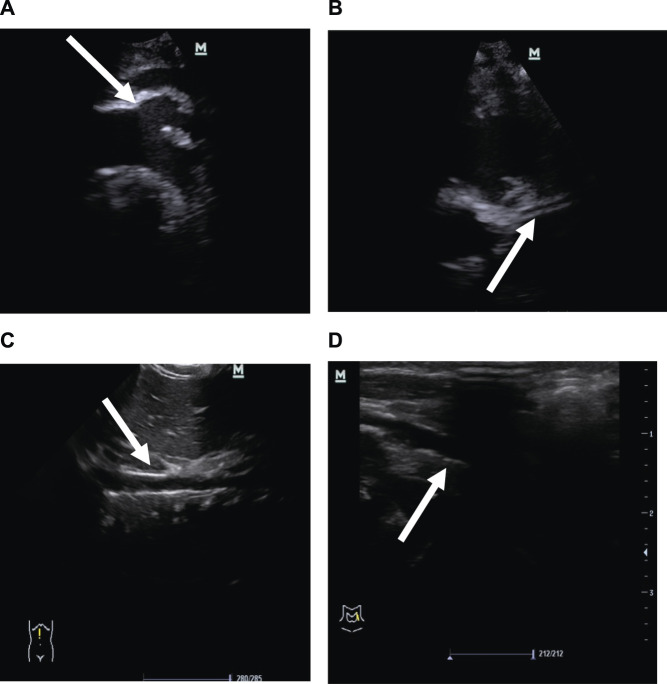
Vascular ultrasound images. (A) Strong echo in the aortic arch and its branches is indicated by the arrows. (B) Strong echo in the left coronary artery wall is indicated by the arrows. (C) Strong echo in the abdominal aortic wall is indicated by the arrows. (D) Strong echo in the femoral artery wall is indicated by the arrows.
